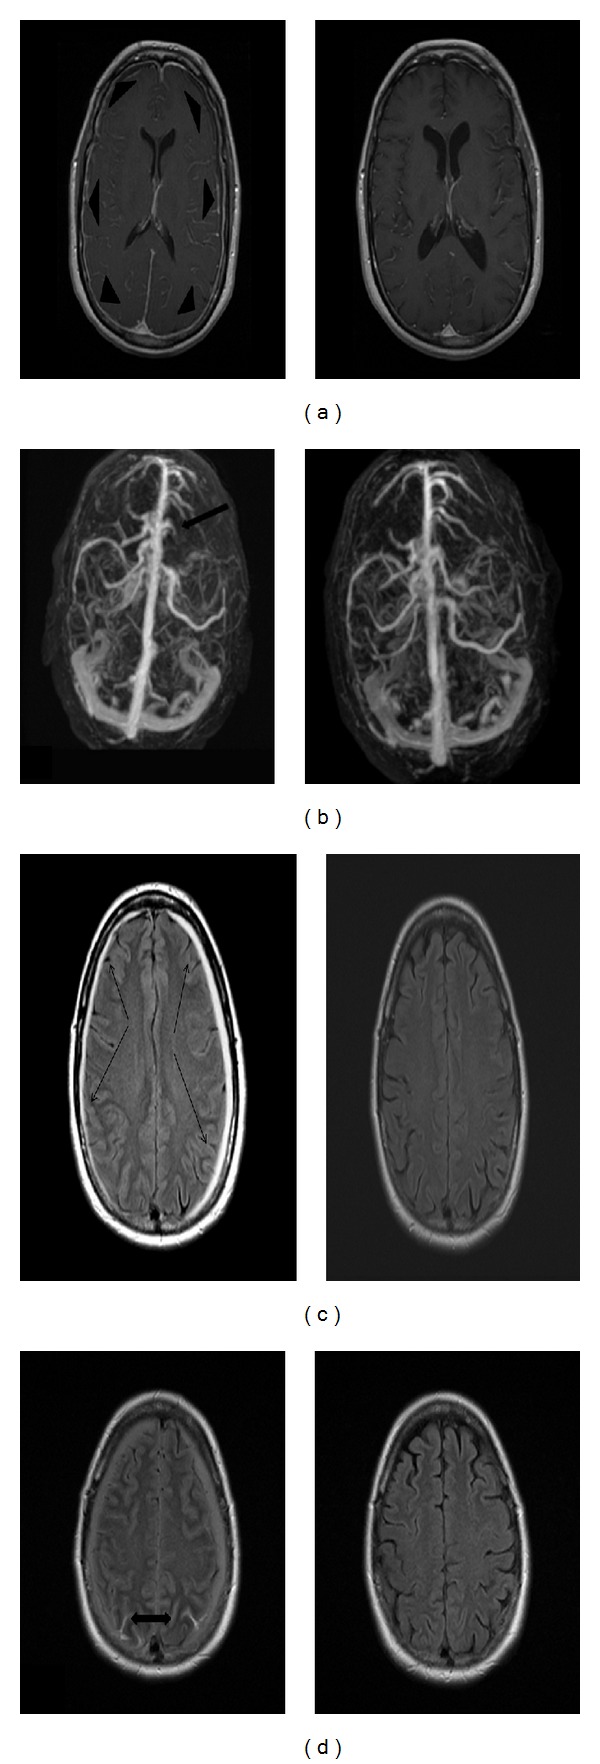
Right side (pretreatment) and left side (posttreatment). Axial T1 weighted magnetic resonance imaging (MRI) after intravenous administration of gadolinium. (a) Brain showing resolution of the pachymeningeal enhancement (arrow heads); (b) magnetic resonance venogram showing occlusion of the left cortical vein (arrow). Axial fluid attenuated inversion recovery MRI brain (c) showing resolution of subdural hematomas (arrows) on the bilateral convexities; subarachnoid hemorrhages (d) in the bilateral parietal lobes (bidirectional arrow).
